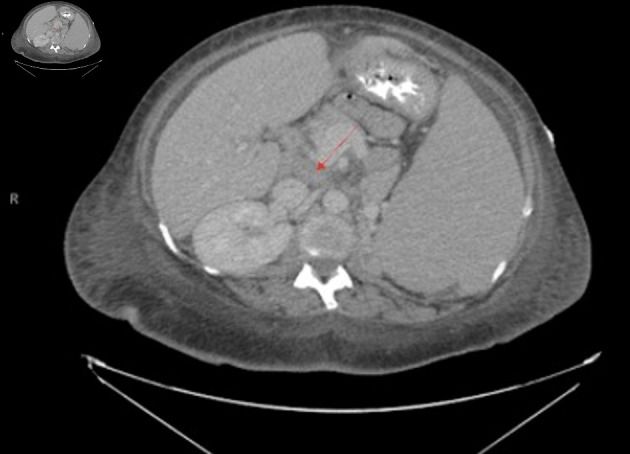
Transverse view of CT chest/abdomen/pelvis showing abdominal lymphadenopathy with noted splenomegaly, with arrow pointing to prominent lymph node. A 3.4 × 2.1 cm nodule on the left adrenal gland is also noted.
